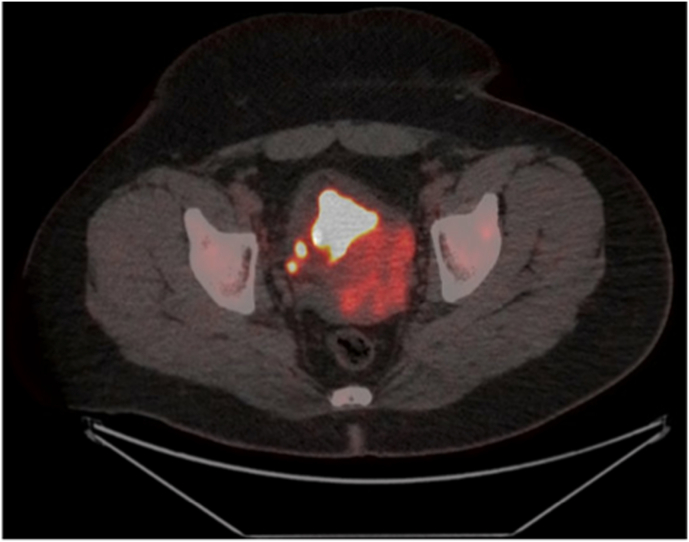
Fused Axial FDG Positron Emission Tomography showing FDG Avidity within Prostate Gland suggestive of High Metabolic Activity.
